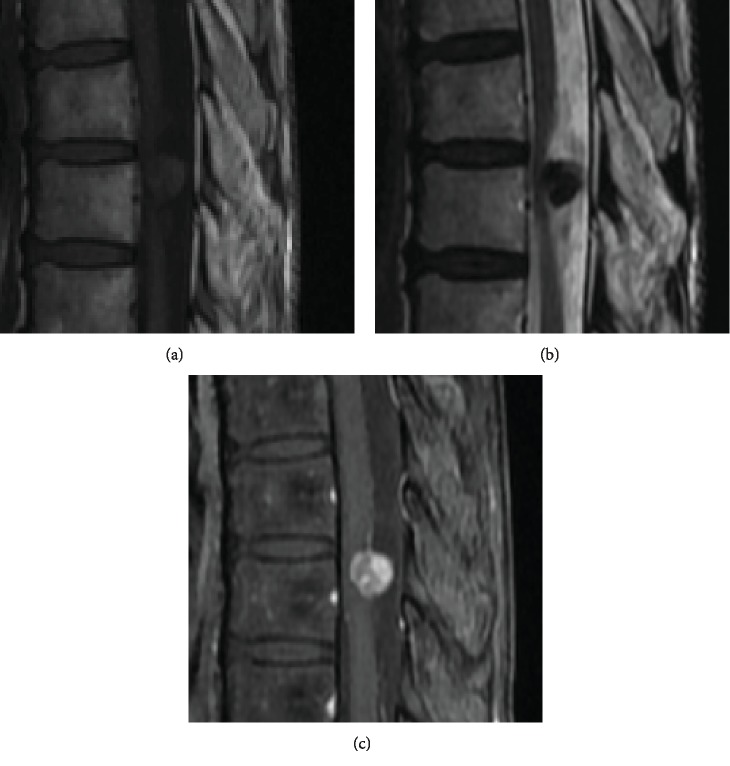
MRI findings showing an intradural-extramedullary mass with isointensity on T1-weighted images (a) and hypointensity on T2-weighted images (b), enhanced heterogeneously with gadolinium (c).
